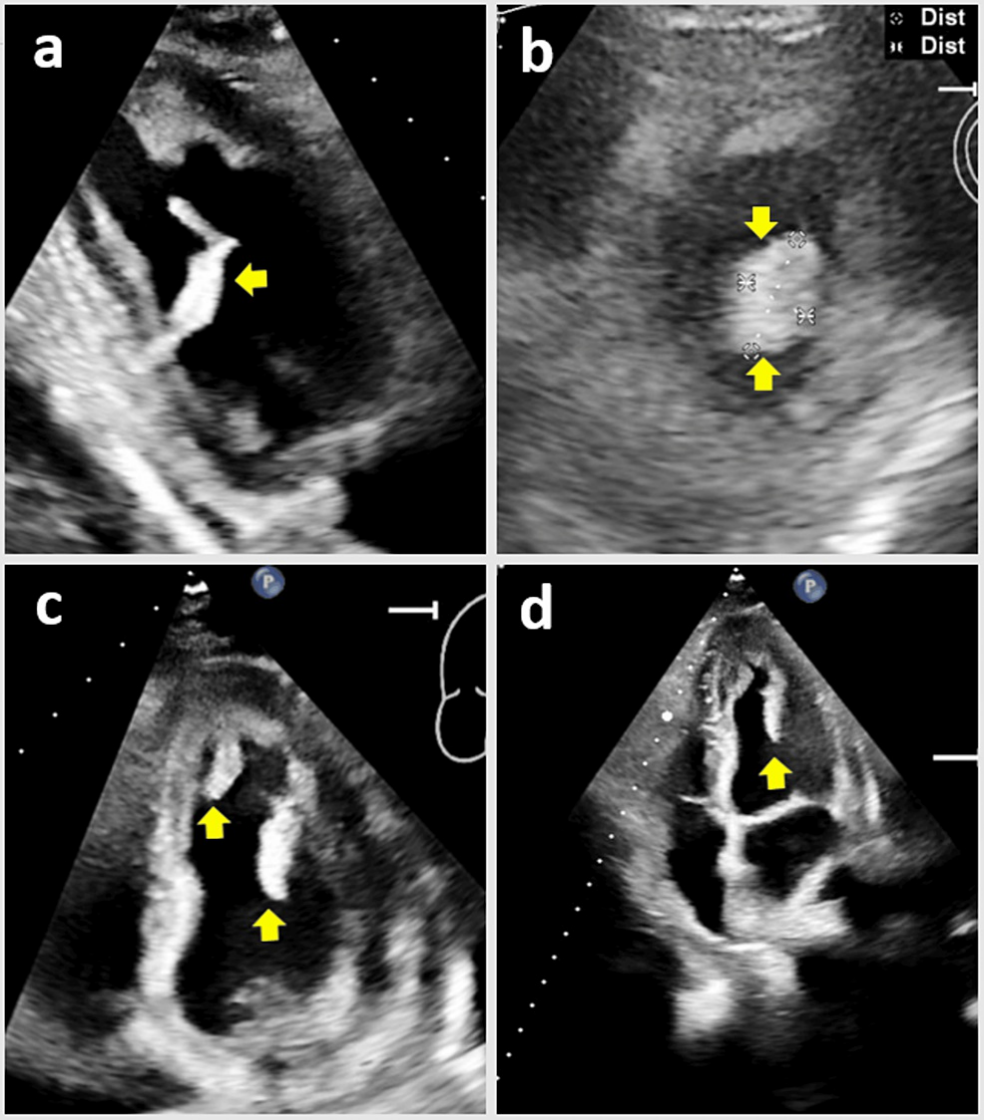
The TTE nine months ago demonstrated intraventricular thrombi when the patient presented with multiple thromboembolic complications (a and b) LV inferolateral wall thrombus on parasternal long and short axis views, respectively. (c and d) Apical and inferolateral floating thrombi on apical four-chamber view TTE, transthoracic echocardiogram; LV, left ventricular
